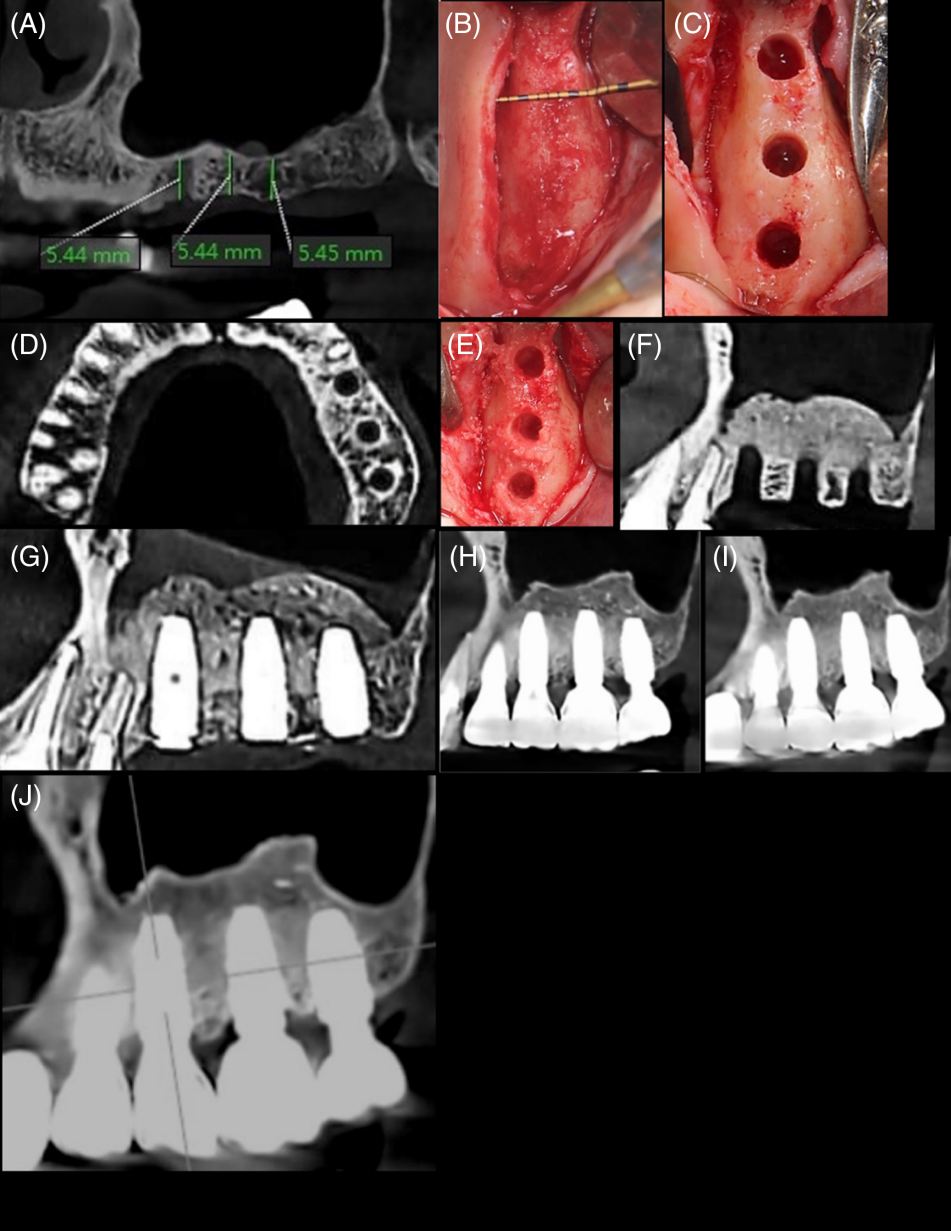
(A) OD Sinus Protocol II (maxillary left quadrant): CBCT scan depicting sinus pneumatization with 5.5 mm alveolar ridge height. (B) Occlusal clinical view of maxillary left quadrant with missing second premolar and upper first and second molar. Adequate alveolar ridge width >5 mm is evident. (C) Occlusal clinical view of three osteotomies preparation with osseodensification. (D) CBCT scan demonstrating the three sperate osteotomies with evident of osseodensification. (E) Occlusal clinical view of three osteotomies preparation with OD crestal sinus grafting protocol II utilizing Allograft. (F) CBCT radiograph demonstrating adequate lift with no evidence of membrane perforation and efficient grafting. (G) CBCT scan demonstrating adequate lift with grafting with no evidence of membrane perforation with simultaneous implants placement. (H–J) CBCT follow‐up of 1 year, 3 years, and 4 years, respectively, demonstrating a stable osseointegration of the three implants placed in the grafted upper left sinus with maintained crestal bone and sinus graft height in area. Clinical case is a courtesy of Dr. Chang WenPing.
